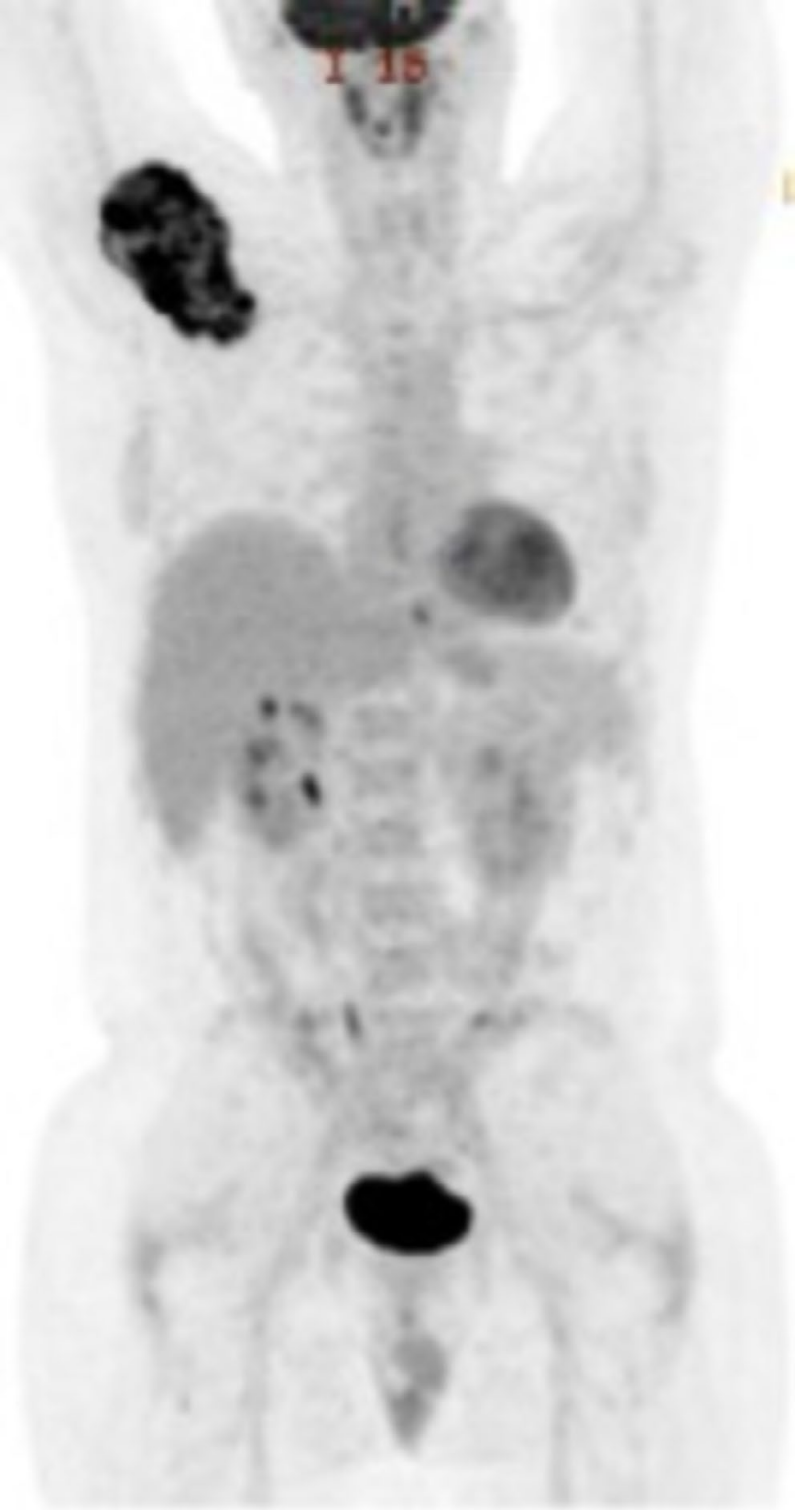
Whole body PET/CT scan depicting oligometastatic disease to the right scapula with no other sites of evident metastasis.
